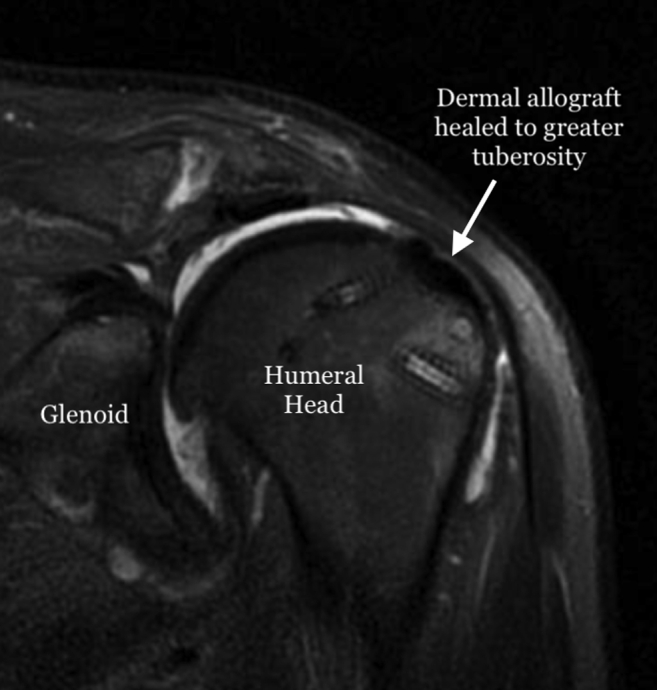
T2-weighted fat-saturated magnetic resonance imaging scan of a left shoulder 3 months after surgery demonstrating healing and incorporation of the graft to the greater tuberosity.
